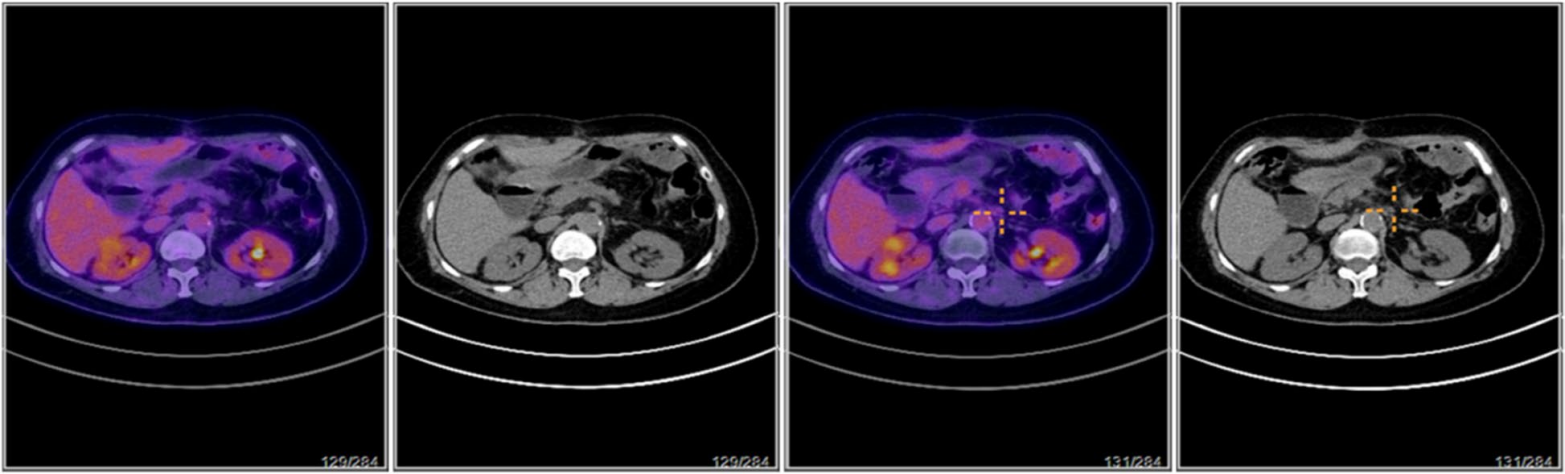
PET/CT Follow-up Examination on April 17, 2023
